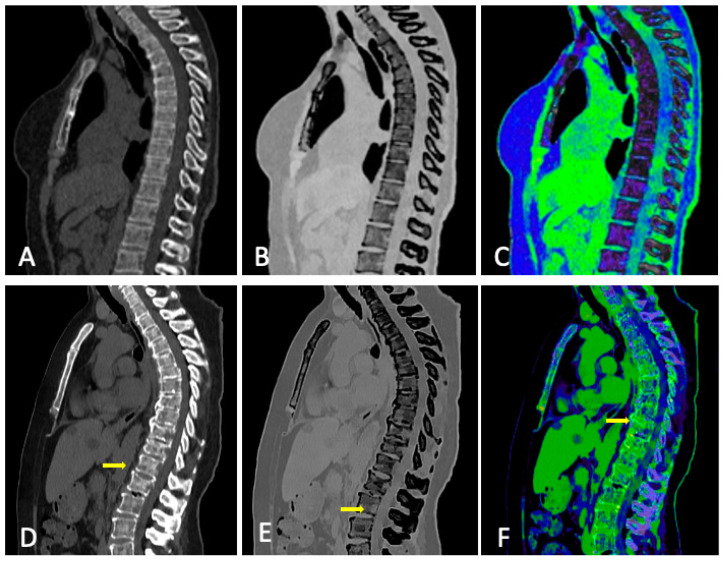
Normal bone (top row) vs. diffuse marrow infiltration with multiple osteolytic lesions (bottom row, arrows) in CT: (A,D) conventional CT, (B,E) grayscale VNCa image, and (C,F) color-coded VNCa image.
